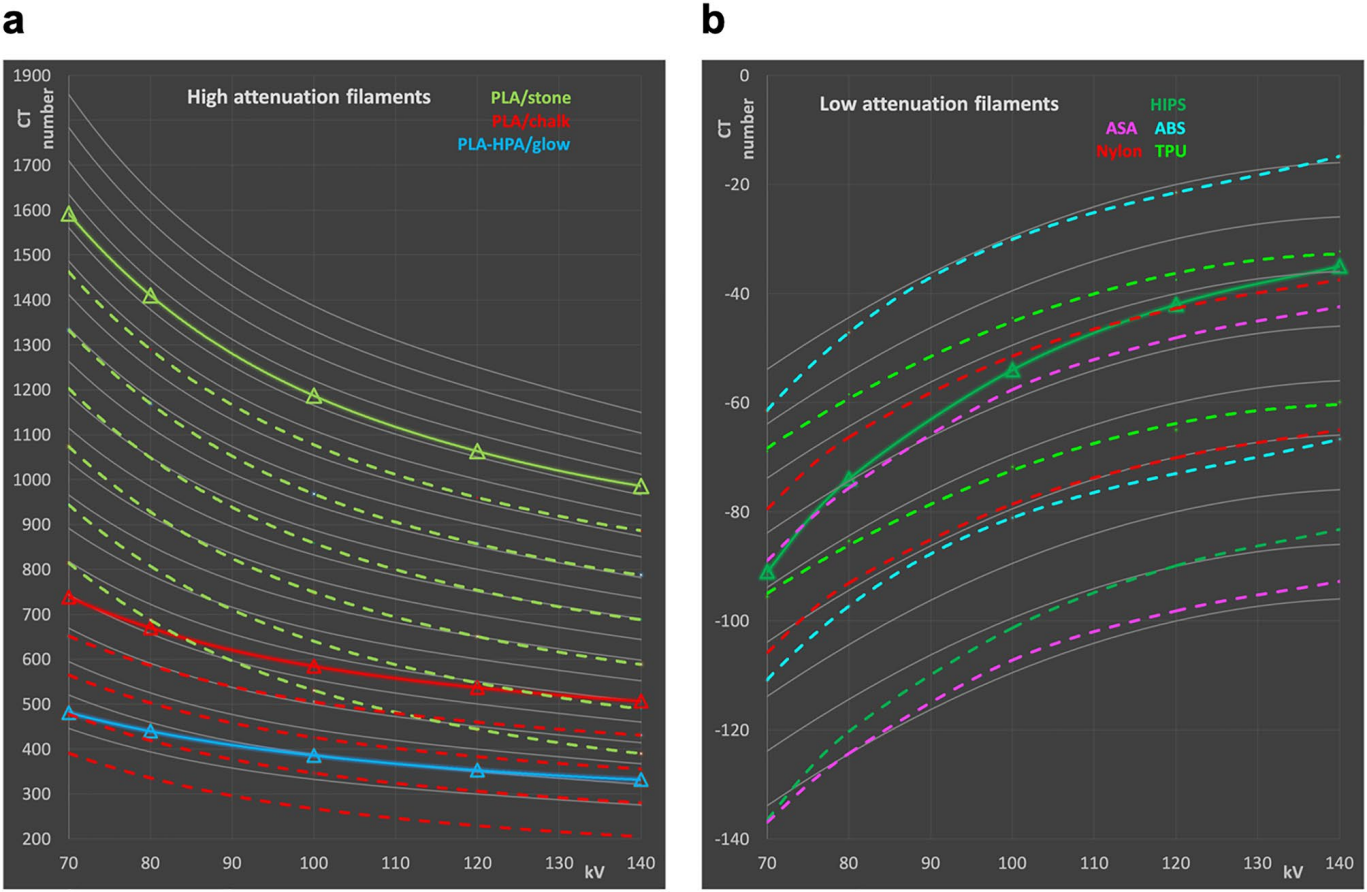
Calculated CT numbers of samples with reduced density (broken lines) compared to bone (a) and adipose tissue (b) in the HU ranges of interest. (a) PLA/stone measured and with reduced density in 5% steps from 95 to 70%; PLA/chalk: measured CT values, and 95–80%, and measured values for PLA/glow. (b) HIPS (100%: measured values, and 95%), ASA (95%, 90%), ABS (95%, 90%), Nylon (87.5% and 85%), and TPU (87.5 and 85%), respectively.
